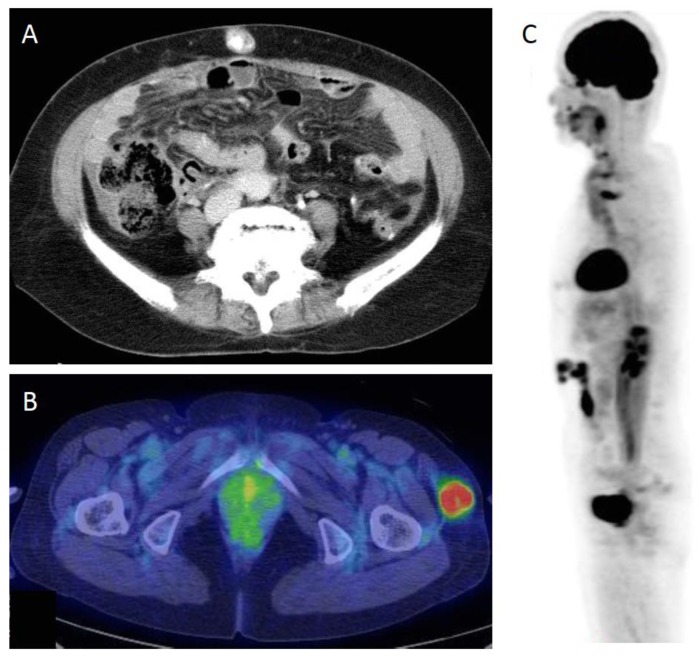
(A) CT scan of umbilical metastasis, a Sister Joseph nodule (SJN). (B) PET/CT scan of a non-SJN metastasis. (C) ^18^F-fluorodeoxyglucose (FDG)-PET image of skin recurrence in surgical scar. FDG uptake is seen in the abdominal surgical scar.
